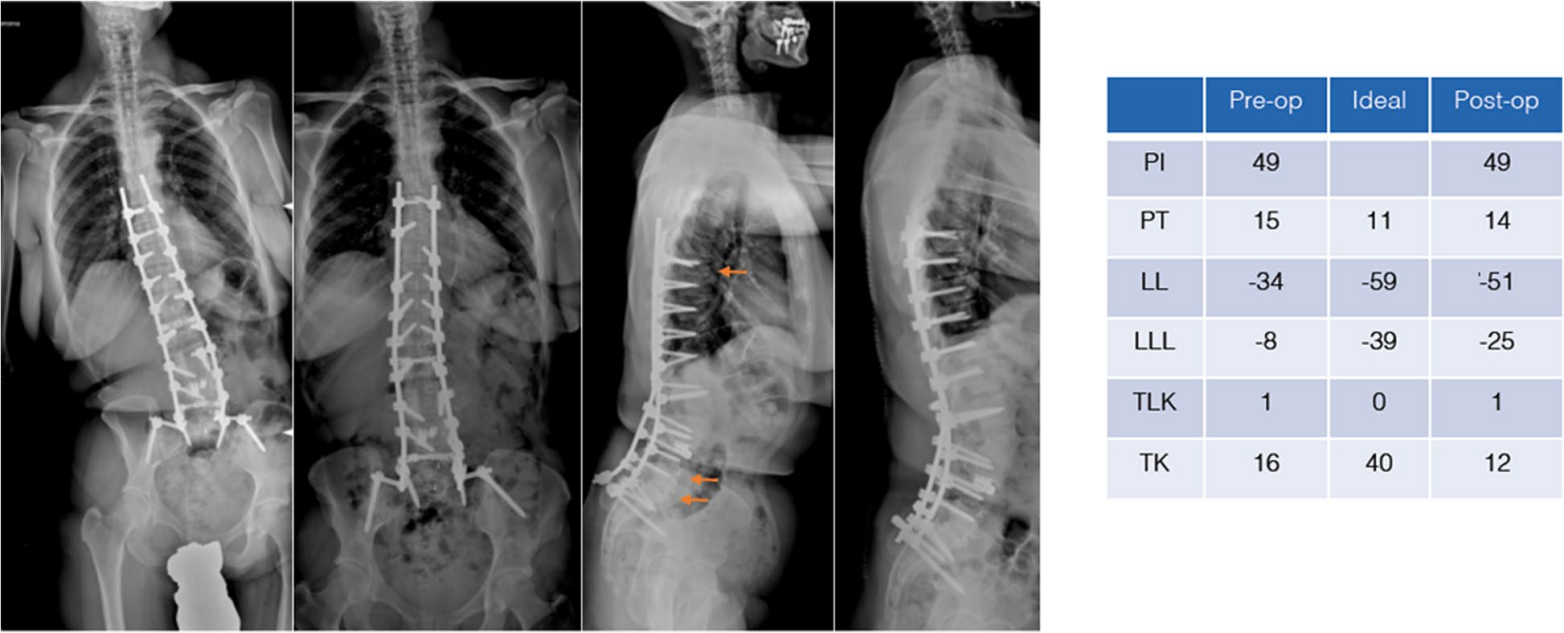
Pre- and postoperative x-ray following revision surgery in March 2013. Before surgery, a T8 fracture and a subsidance of the TLIF cages had been diagnosed (arrows). A T7-pelvis revision with implant renewal was performed. Again, the postoperative sagittal parameters were comparable with normative values with the exception of TK
